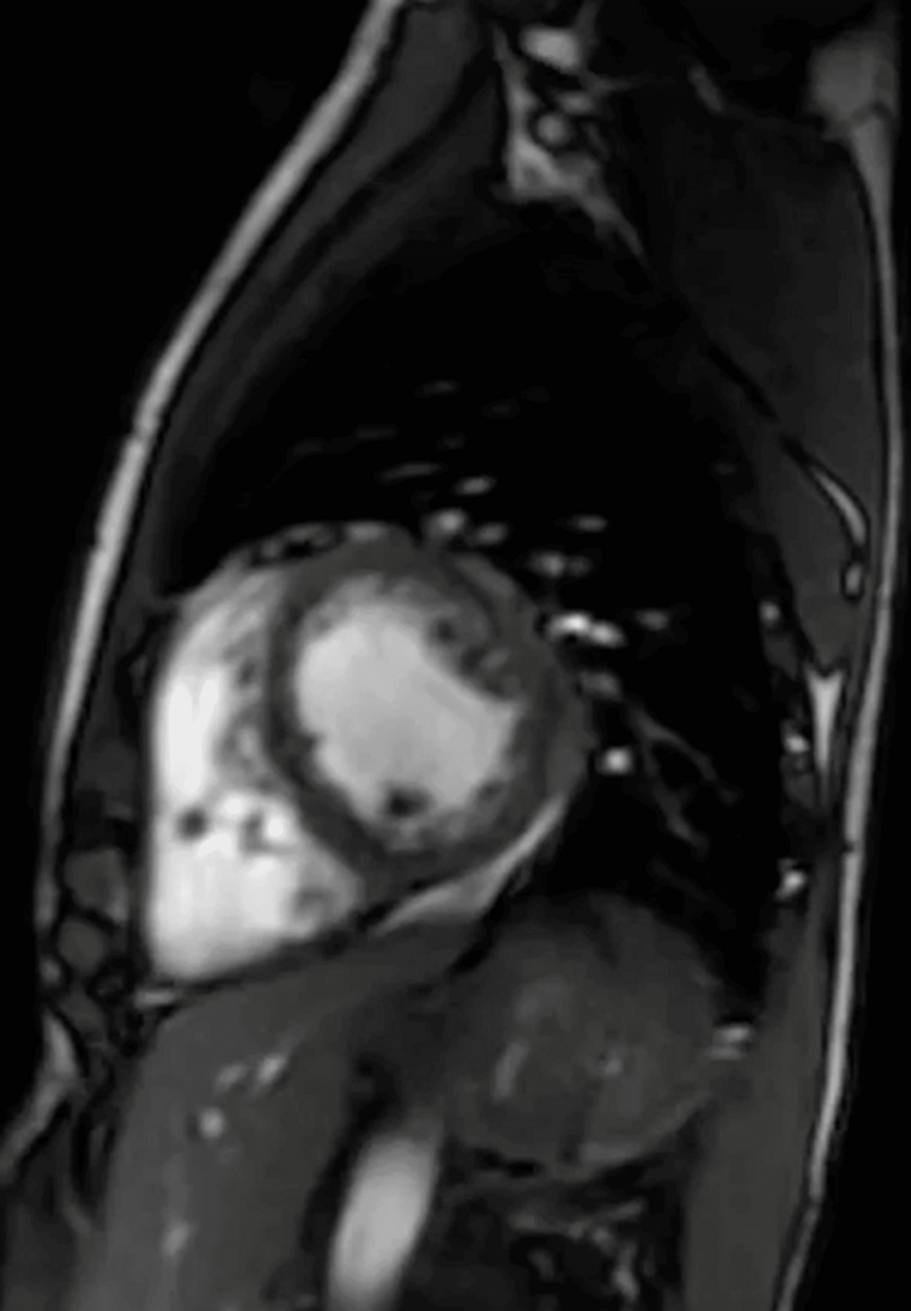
Cardiac MRI short axis view without abnormal late gadolinium enhancement or abnormal signal to suggest myocarditis or infiltrative cardiomyopathy
